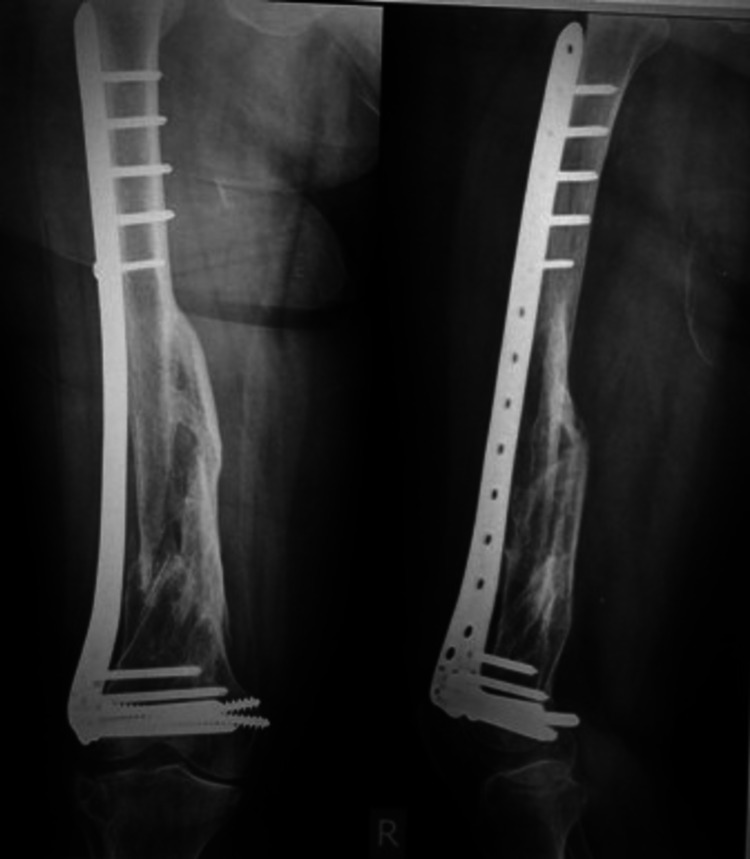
Six months post-operative x-rays showing bridging callus formation. Six months follow-up x-rays show bridging callus with satisfactory alignment in both antero-posterior and lateral views with no signs of implant failure.
